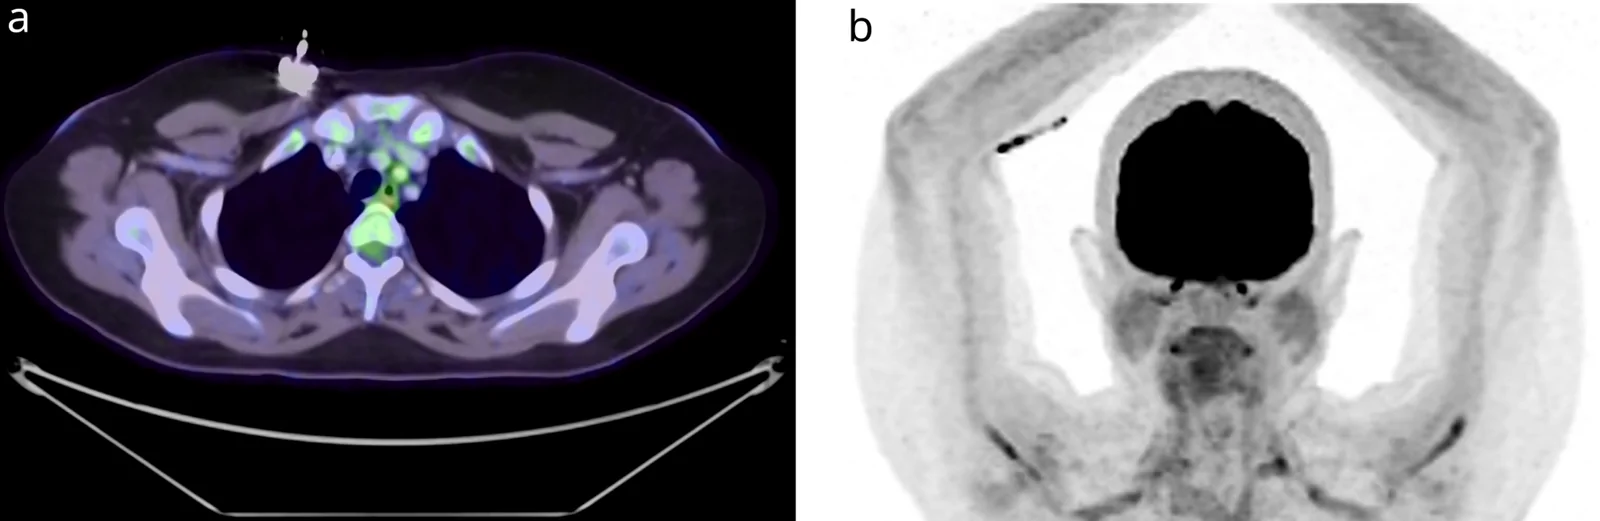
Patient images showing [^18^F]FDG residual in a) an implantable venous port and b) a peripheral intravenous catheter. In both cases, the residual activity was measured to be < 0.05 MBq
